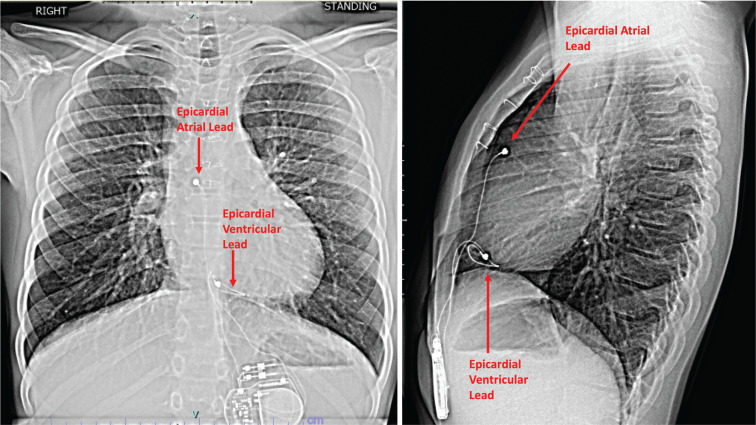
The postero-anterior and lateral chest X-ray with epicardial right ventricular pacing system 2 years prior to presentation. Note the right ventricular basal location of the ventricular lead.
